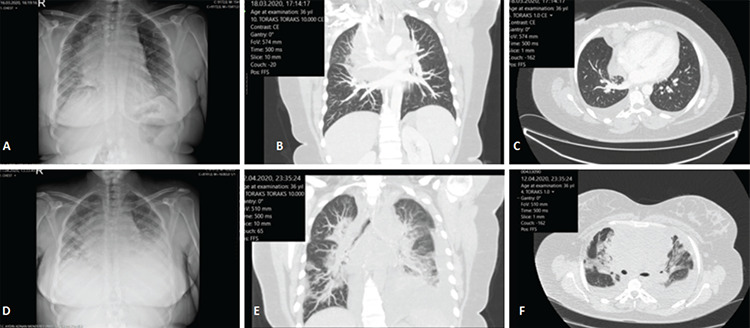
A computed tomography scan revealed a mediastinal mass surrounding the aorta and supraaortic vascular structures, compression of the superior vena cava, collapse and consolidation in right lung, and bilateral pleural effusion.
